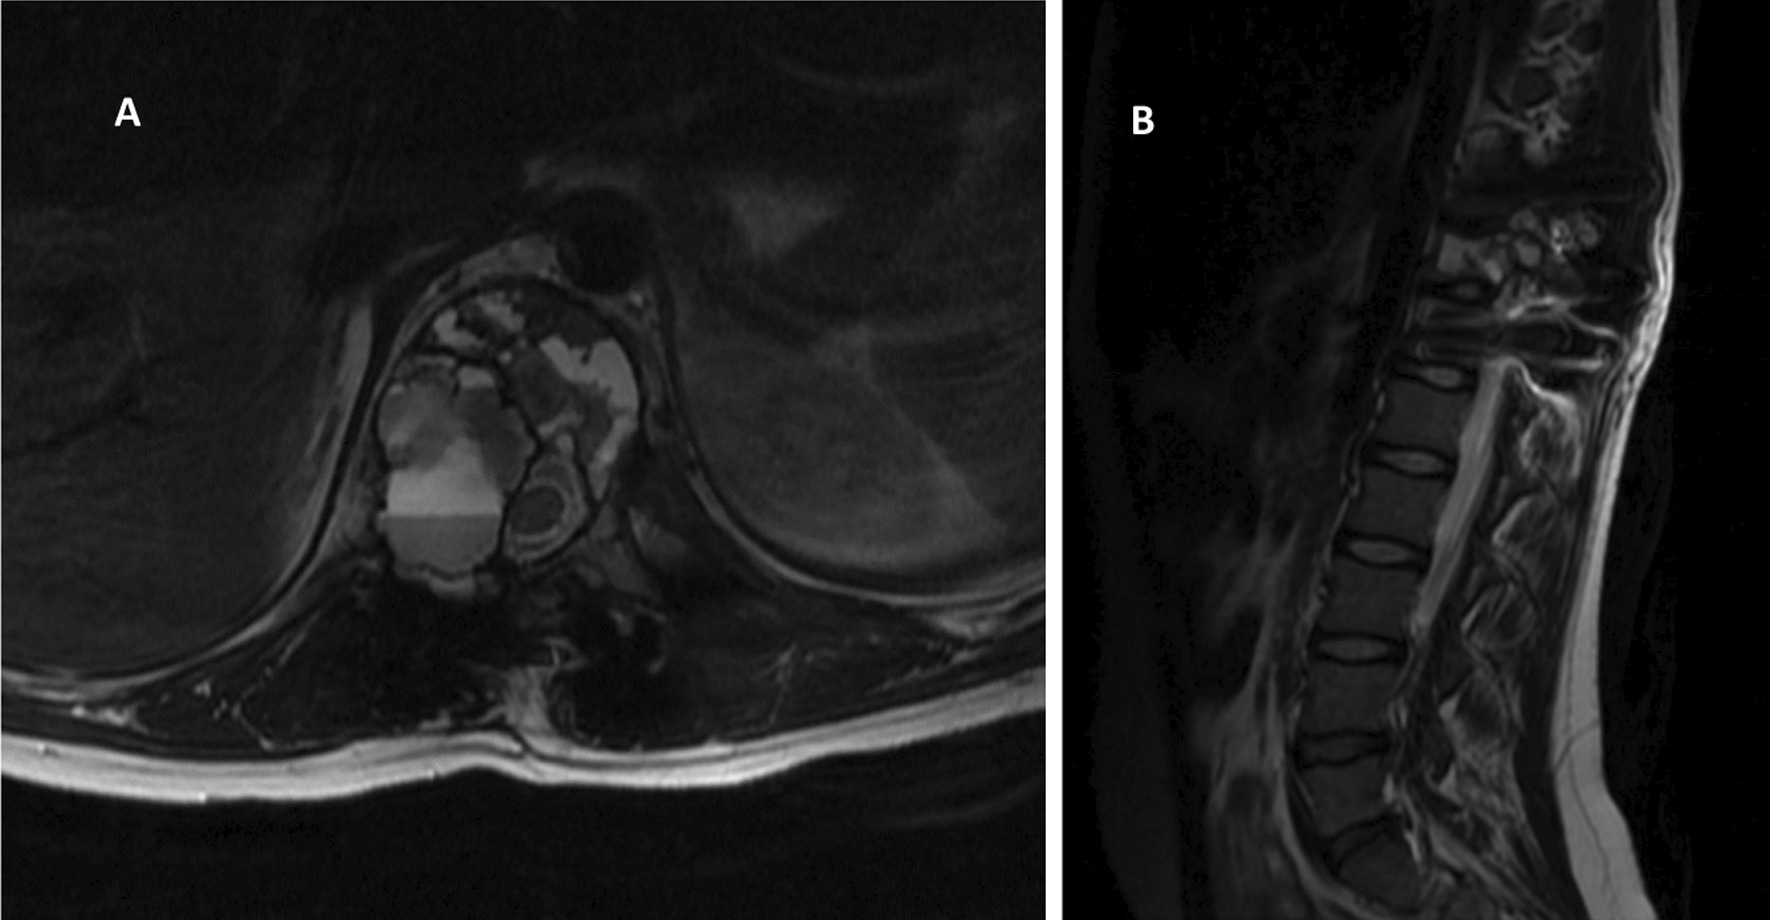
A, B MRI A axial and B sagittal showing Expansile bone lesion with fluid–fluid level at T11, keeping with ABC causing moderate compression fracture with extension into posterior elements causing sever right foraminal stenosis and mild cord compression
